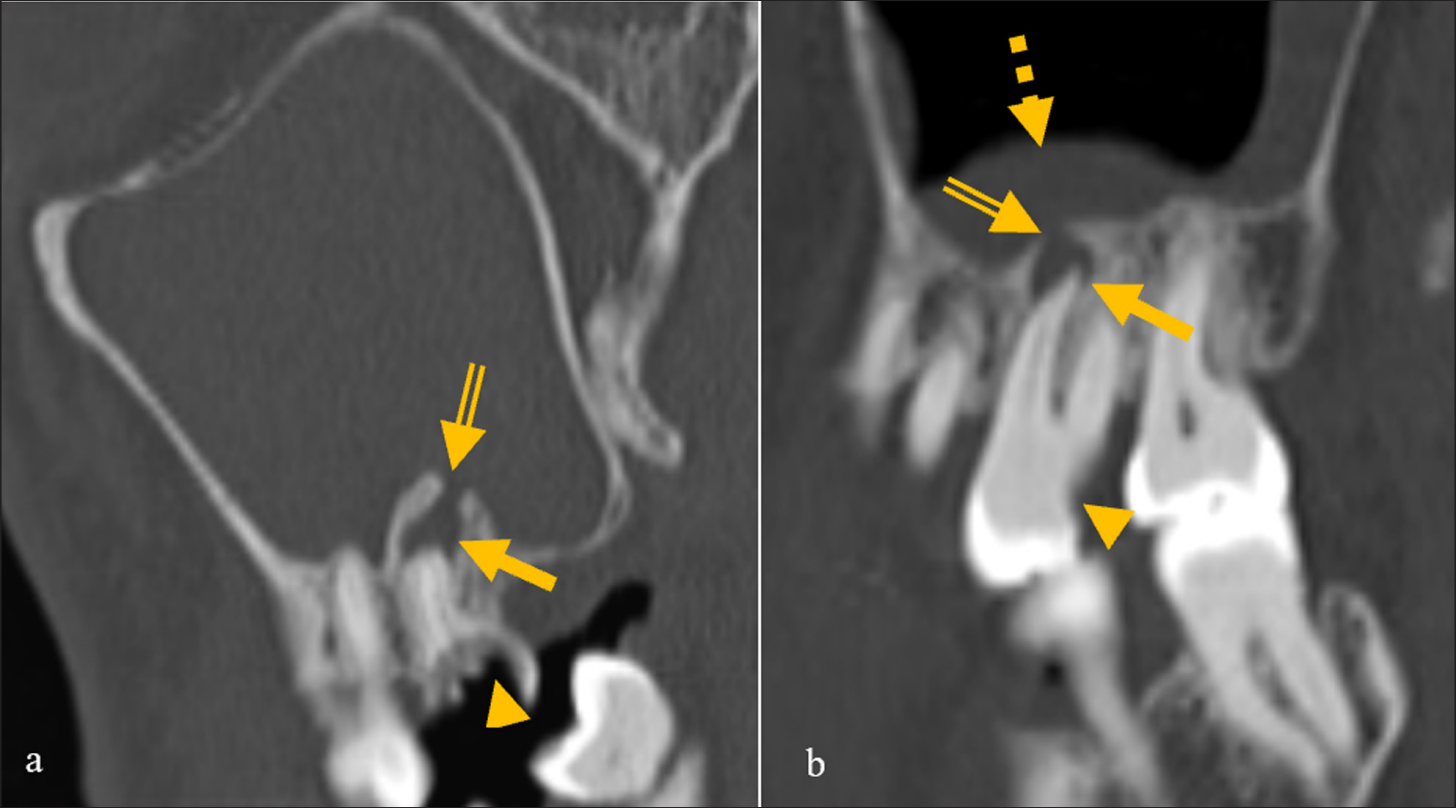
Images of two different patients showing dehiscence of the floor of the maxillary sinus (double lined arrow) and mucosal thickening and the causative diseased tooth with caries (arrowhead) and periapical lucency (arrow).
